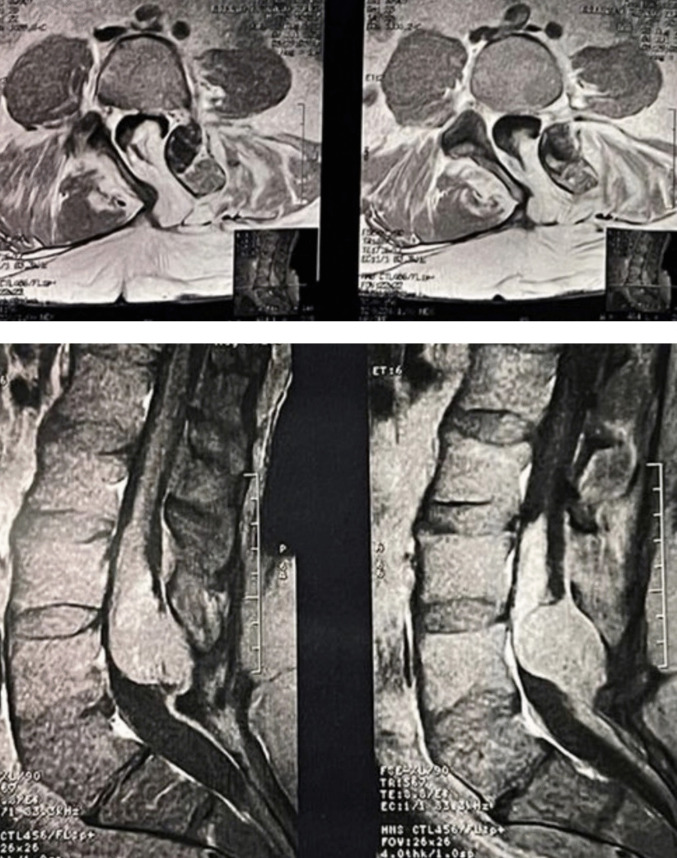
Lumbar spine MRI—preoperative evaluation
